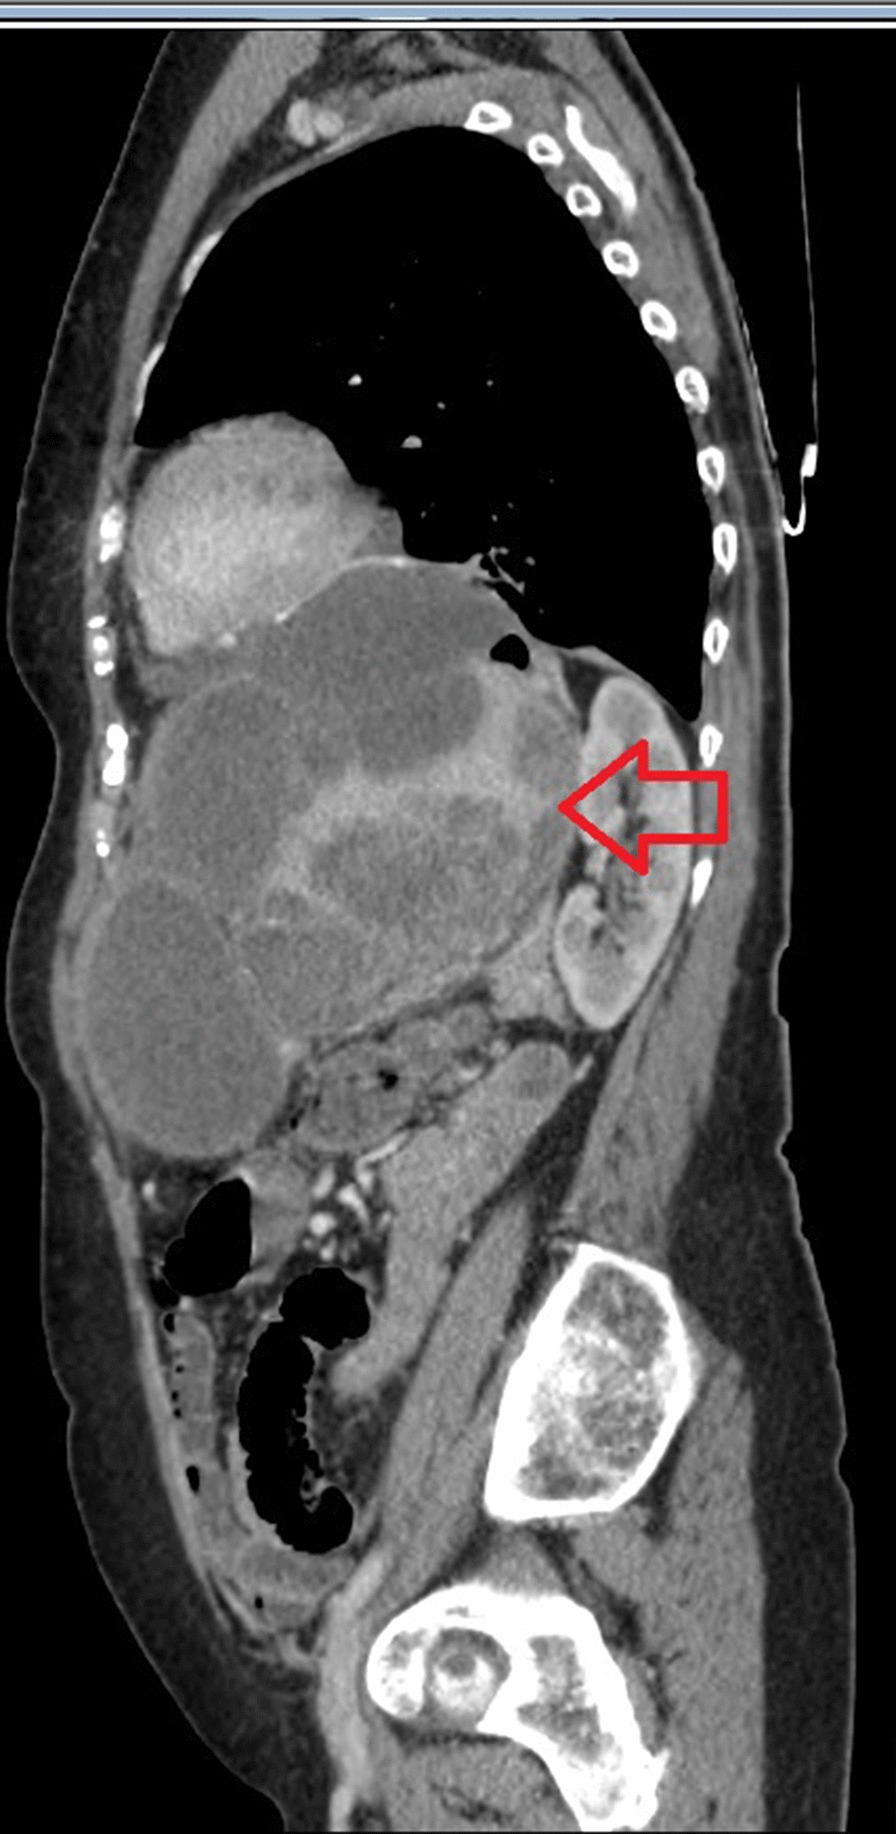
CT-sagittal view of recurrent tumor at the site of the spleen, 3 year after surgery (red arrow)
